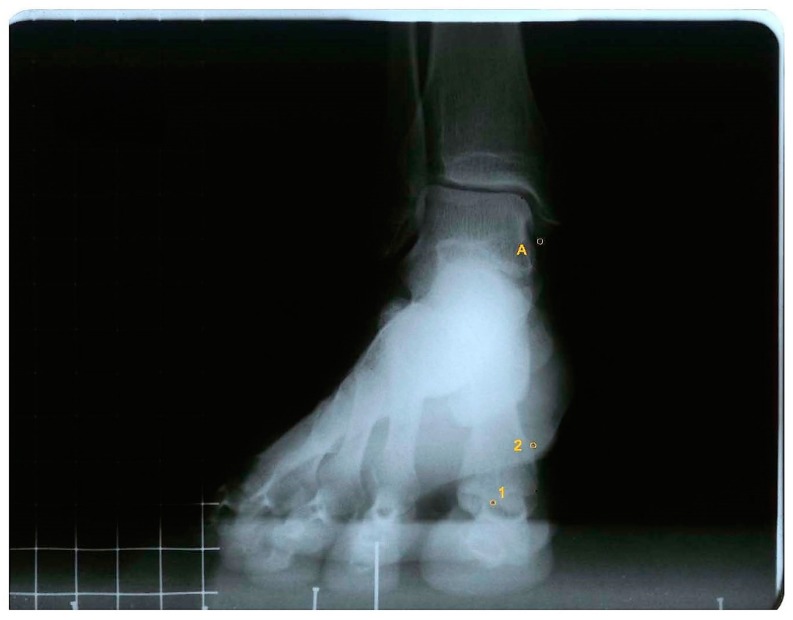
Antero-posterior radiograph of a participant with the first ray in a neutral position, showing point A (most distal point of the tibial malleolus), point 1 (intersesamoid crest), and point 2 (superomedial tubercle of the head of the first metatarsal).
